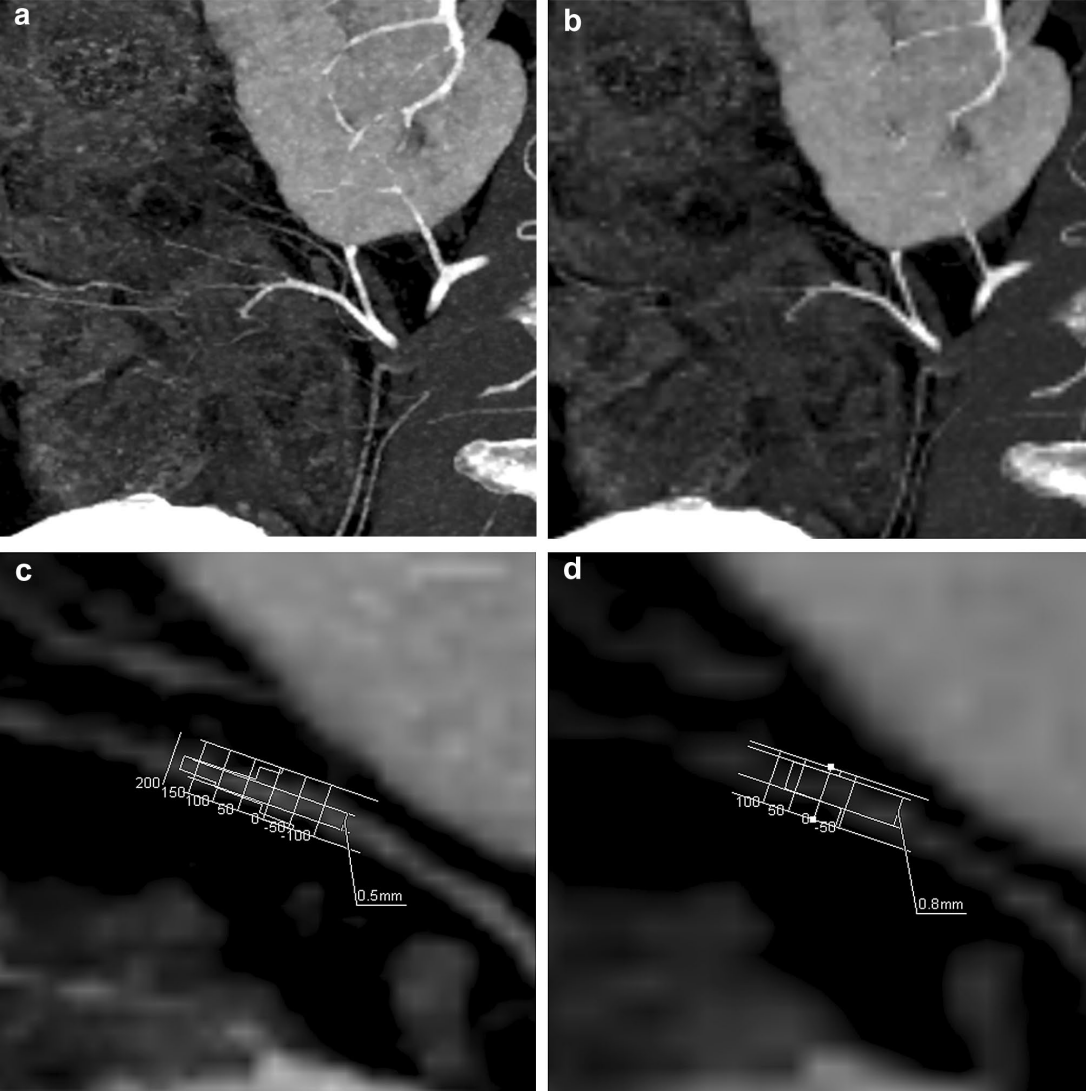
Partial MIP image of CTA of SMA branches. a : HR CTA with a matrix of 1024 × 1024 more accurately delineated the marginal arteries and vasa recta than (b): NR CTA with a matrix of 512 × 512. Profile curves of the small mesenteric artery of (c): HR CTA and d: NR CTA
